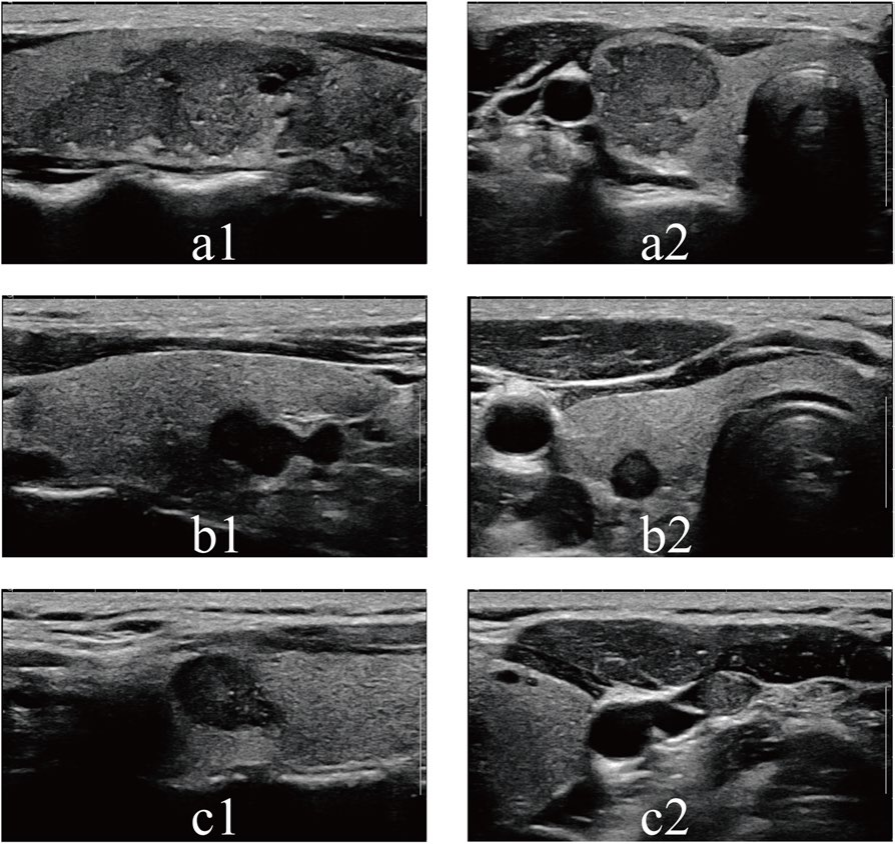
Ultrasound features of the PTC tumor and lymph node: a1 and a2 show tumor size > 2 cm and capsule contact; b1 and b2 show extrathyroidal extensions (ETE); c1 and c2 show capsule contact and lateral metastatic lymph node (III): the lymph node appear enlarged, round
